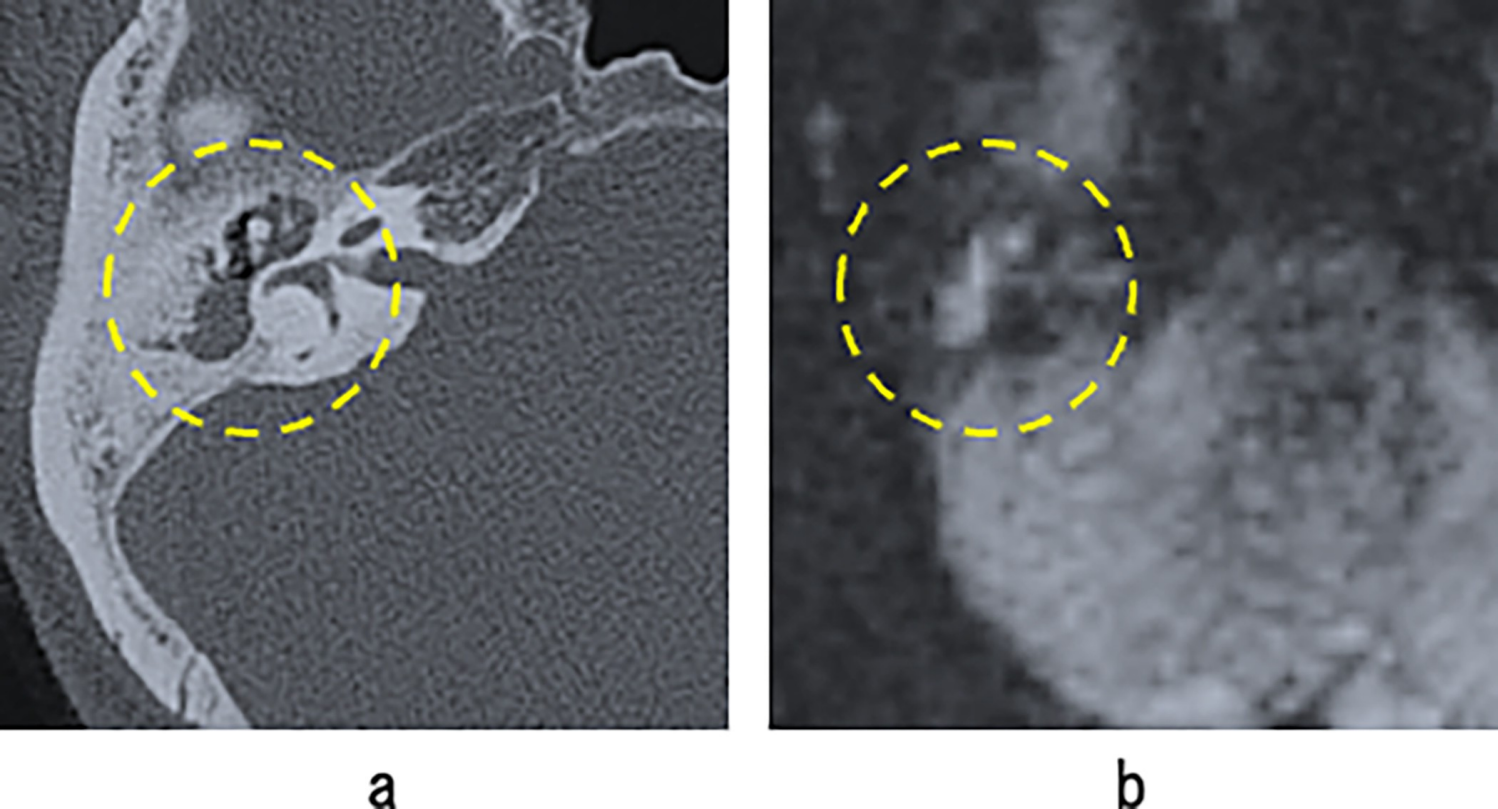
CT and MRI (non-EP diffusion-weighted imaging) findings in patients with cholesteatoma. Yellow dotted lines indicate lesions. (a) CT showing a soft density lesion from the attic to the mastoid. It is difficult to distinguish between cholesteatoma and non-cholesteatoma inflammatory lesions. (b) MRI can diagnose the condition but the exact extent is difficult to determine.
